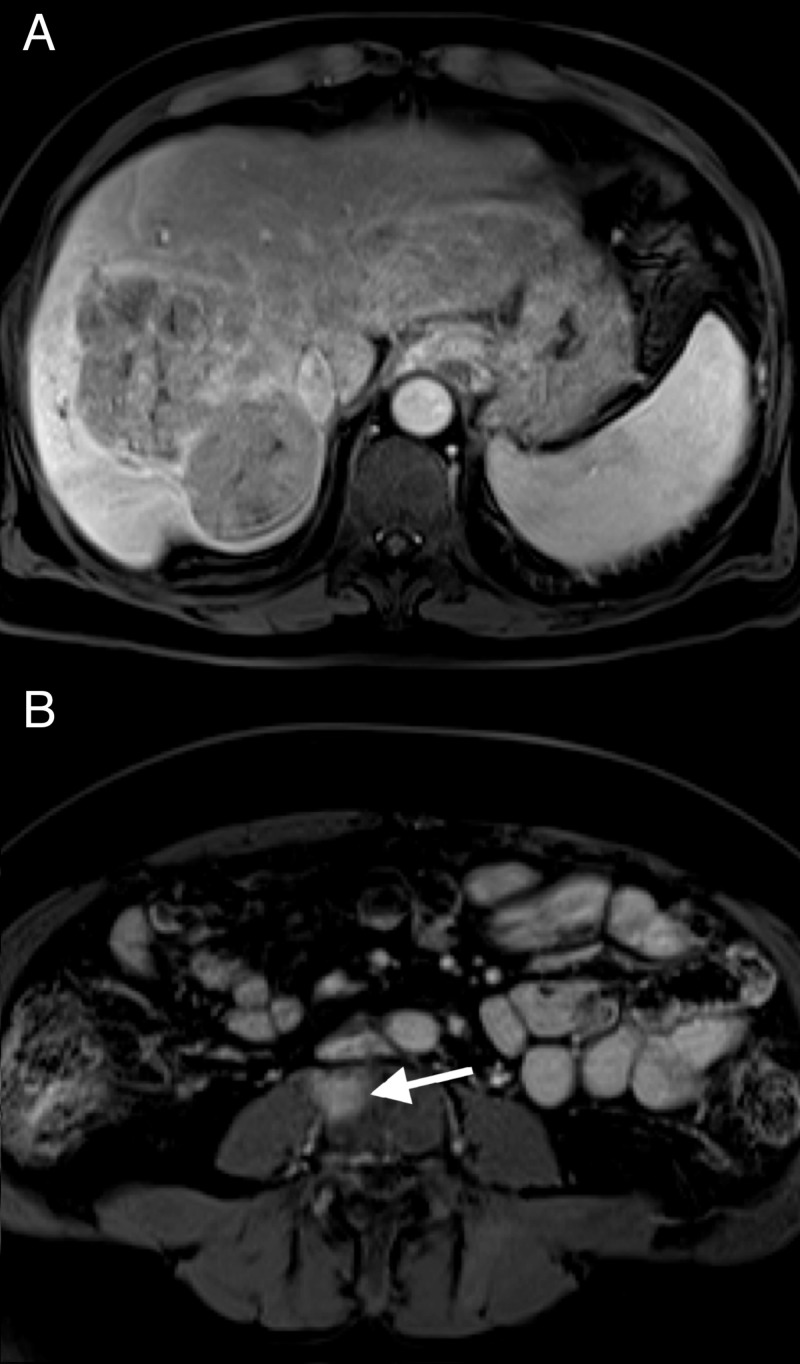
September 2017 MRI with IV contrast arterial phase 20-second (T1) vibe sequence (A) Reduced vascularity of right hepatic lobe tumor; (B) new L4 lesion MRI: magnetic resonance imaging, IV: intravenous
